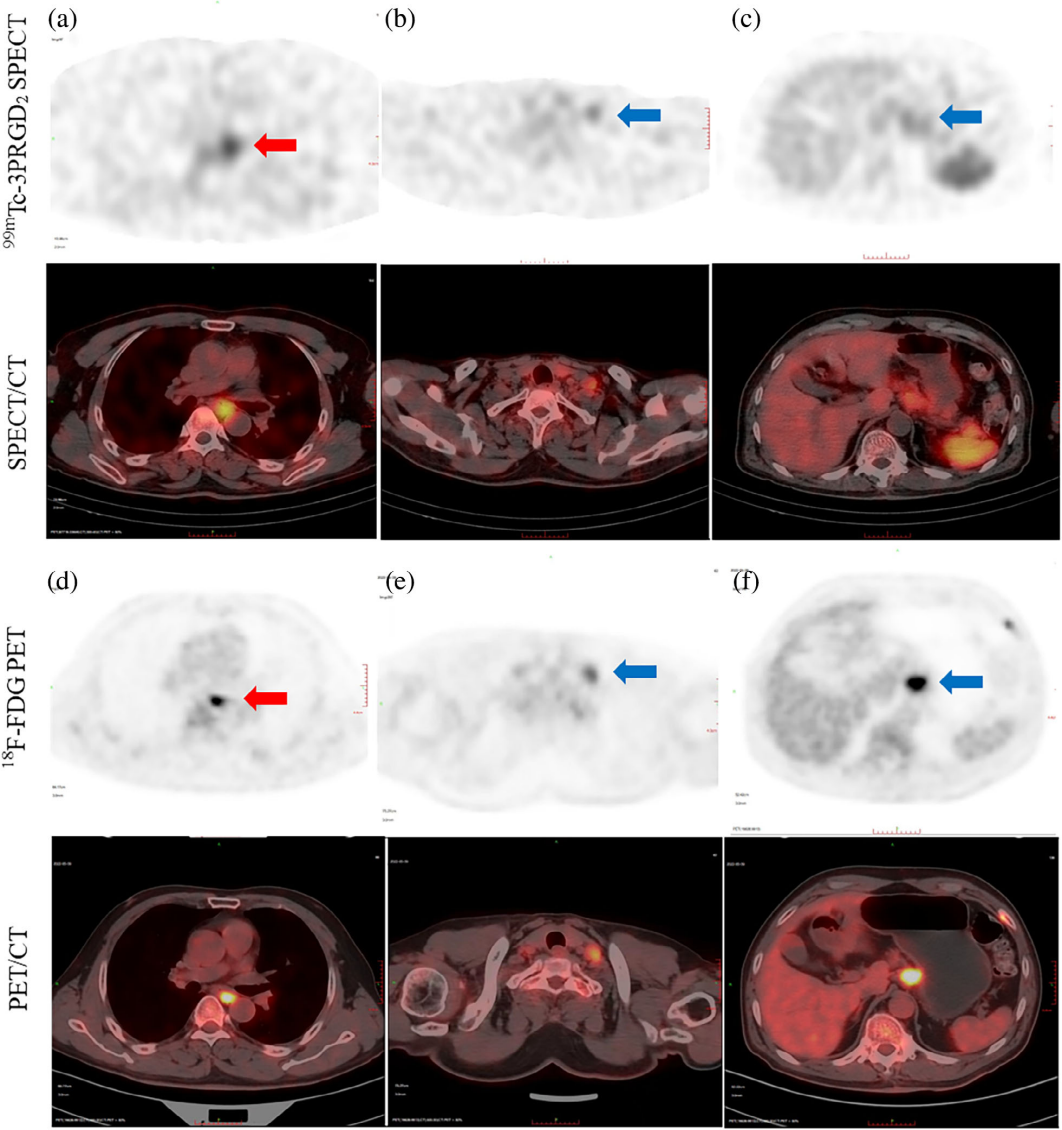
Representative images of ^99m^Tc‐3PRGD_2_ single‐photon emission computed tomography (SPECT/CT) and 18F‐fluorodeoxyglucose positron emission tomography‐computed tomography (^18^F‐FDG PET/CT). A 69‐year‐old man with esophageal squamous cell carcinoma (ESCC) and lymph node metastasis. (a) The SPECT scan demonstrating increased ^99m^Tc‐3PRGD2 uptake (SUV_max_ = 3.6) in the primary lesion. (b, c) The SPECT/CT scan demonstrating metastatic lymph nodes (No. 1L and No. 17) with increased ^99m^Tc‐3PRGD_2_ uptake (SUV_max_: 3.1; 2.9). (d) The PET scan demonstrating increased ^18^F‐FDG uptake (SUV_max_ = 3.9) in the primary lesion. (e, f) The ^18^F‐FDG PET/CT scan demonstrating metastatic lymph nodes (No. 1L and No. 17) with increased FDG uptake (SUV_max_: 6.8; 11.3). The red arrows point to the primary lesions of ESCC, and the blue arrows point to the metastatic lymph nodes.
